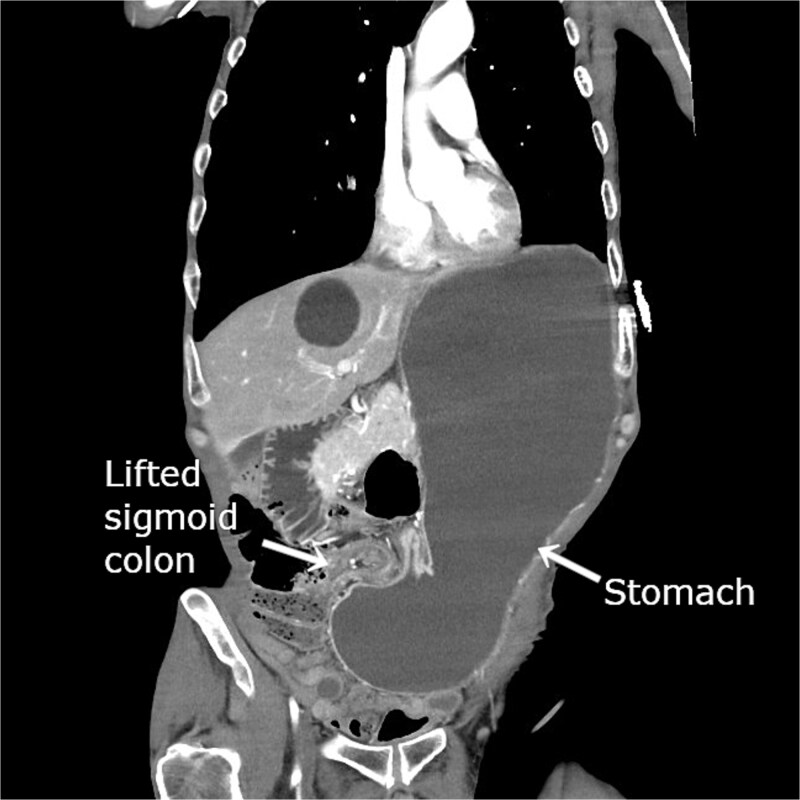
Contrast-enhanced CT revealing gastric herniation through the lateral defect between the lifted sigmoid colon and abdominal wall. The elevated sigmoid colon created for colostomy compresses the pyloric region from the patient’s right side, causing focal narrowing near the antrum. Marked gastric dilatation with significant fluid retention is evident.
